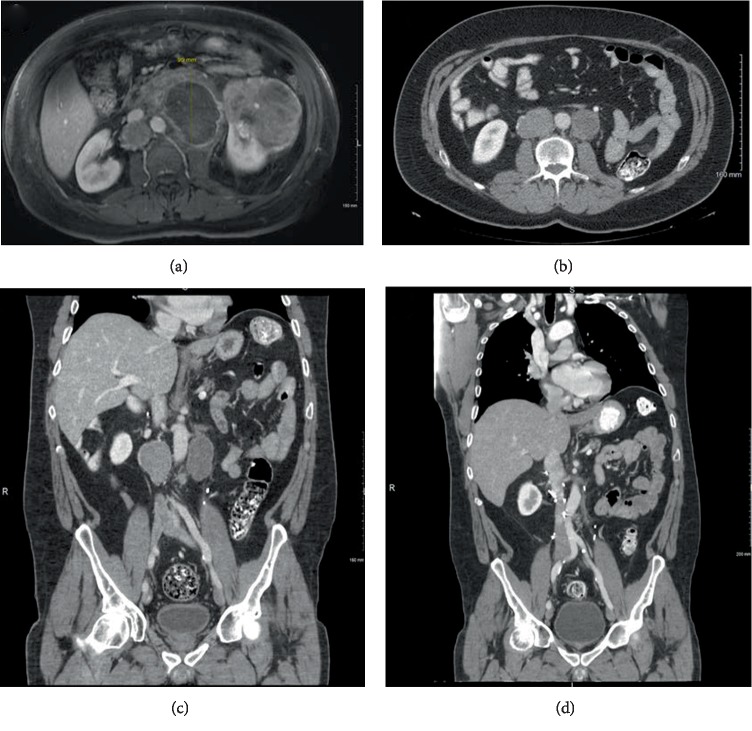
Magnetic resonance imaging (MRI) showing the large renal mass and metastatic lymph nodes. Dramatic reduction in tumor volume in an enlarged lymph node as demonstrated by MRI taken at initial presentation (a) vs. after 5 months of sunitinib (b) and (c). After retroperitoneal lymph node dissection, a complete response was obtained (d).
